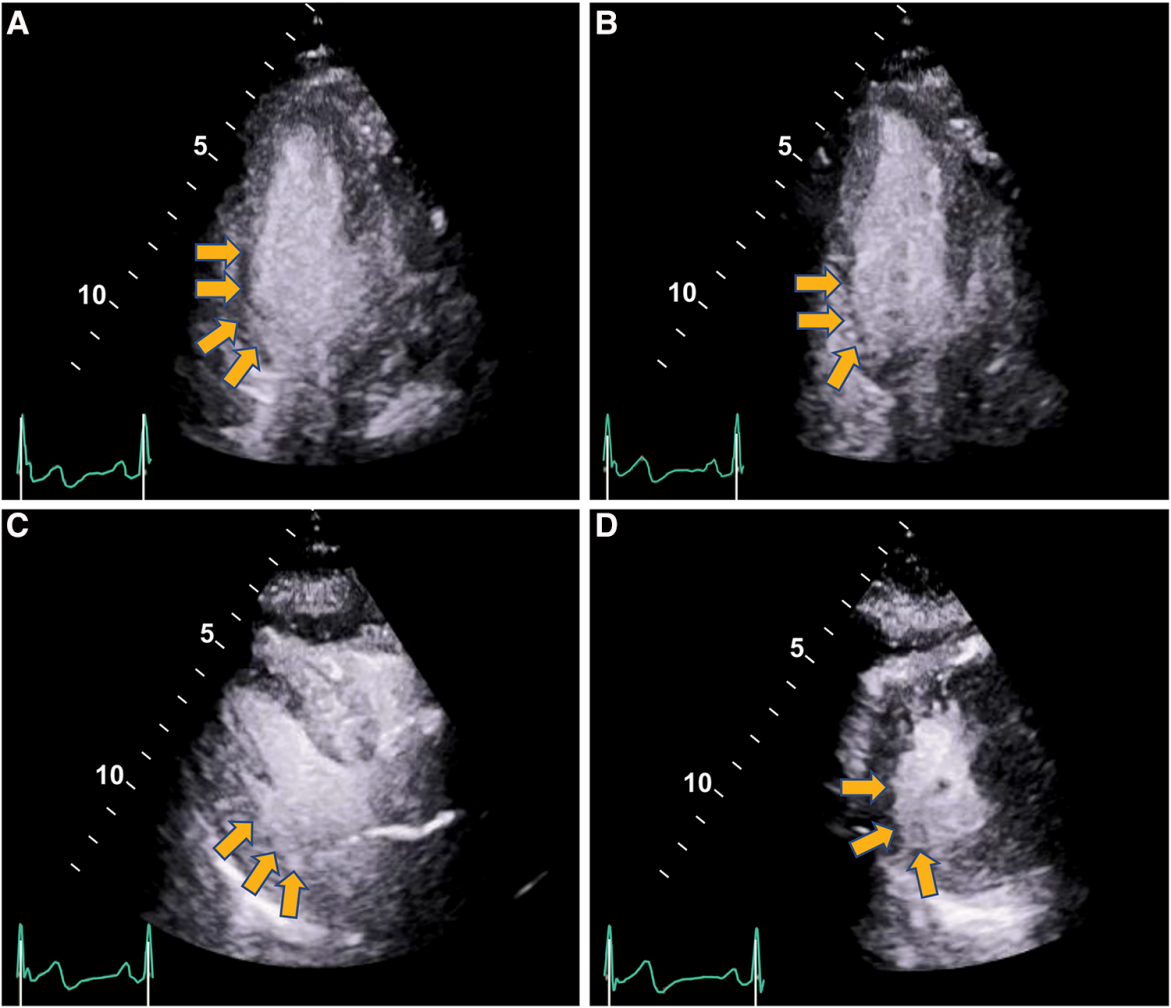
Regional wall motion abnormalities with severe hypokinesis of the basal infero-septal, basal, and mid inferior and infero-lateral segments seen on stress echocardiography during anginal episode (yellow arrows) (end-systolic frames shown). Panel (A) Apical 4-chamber. Panel (B) Apical 2-chamber. Panel (C) Parasternal long axis. Panel (D) Parasternal short axis. (Supplementary Videos S1, S2).
